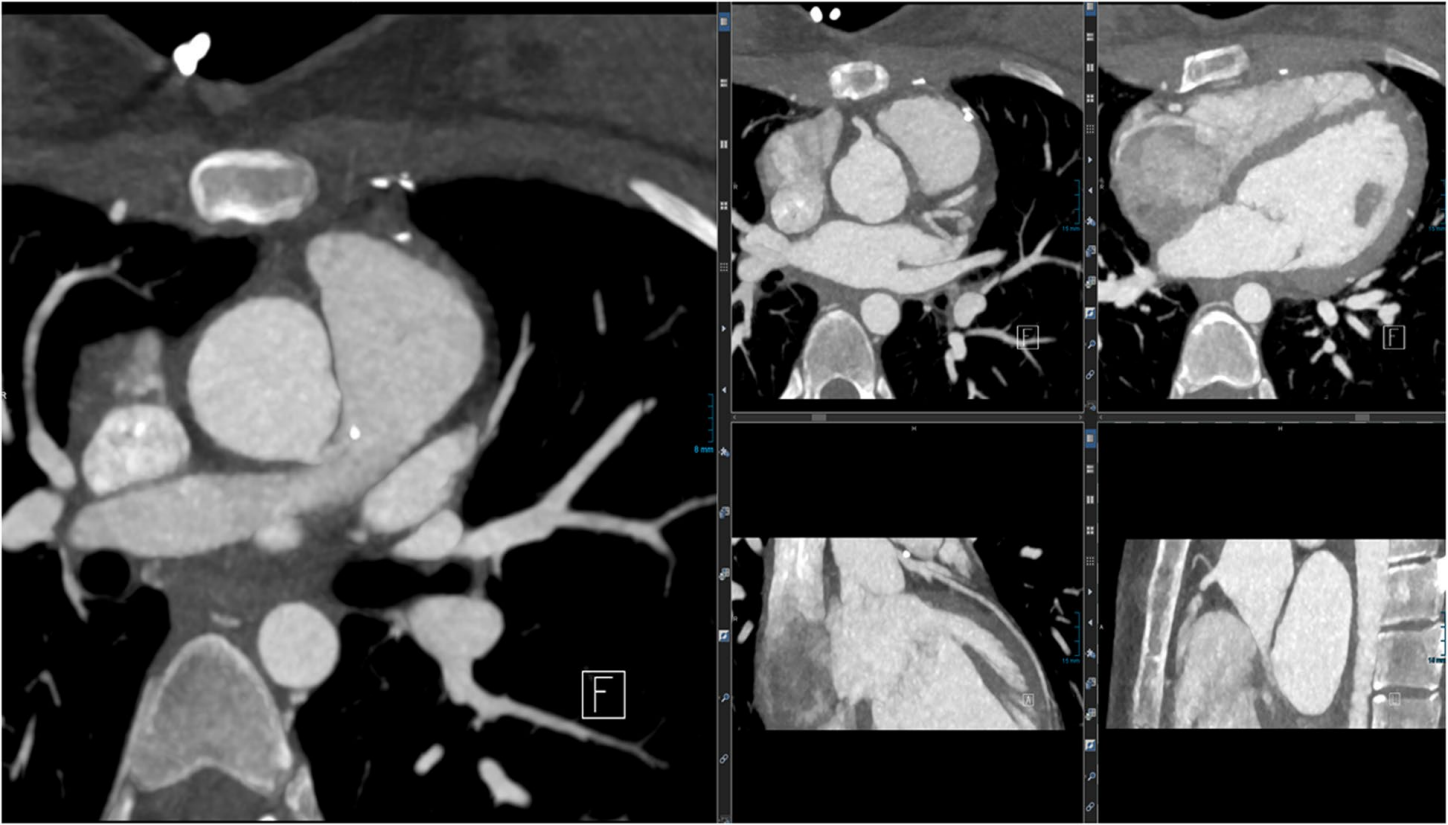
(Representative images for the primary CT datasets): based on primary axial planes (left), different positions in long-axis heart orientation (right upper row) and parasagittal orientation (right lower row) are depicted. Currently, these standard projections are reconstructed by specifically trained technicians; increasingly AI-based algorithms take over these time-consuming tasks
